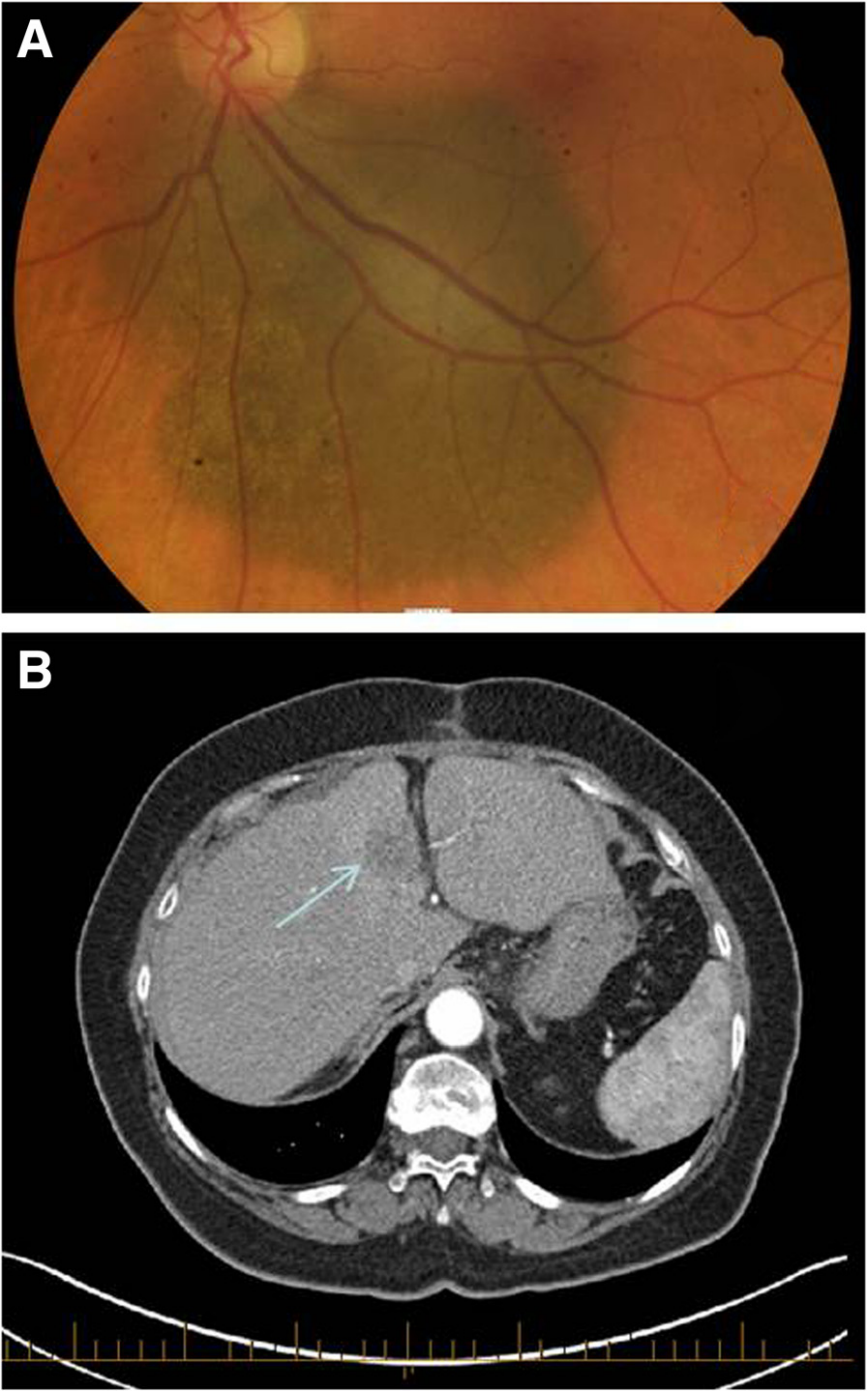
Imaging of patient with germline BAP1 mutation. a. Retinal examination revealed a melanocytic tumor with irregular margins involving the majority of the retina. b. CT of the abdomen prior to surgery identified a 32 mm lobulated lesion in segment 4B of the liver as indicated by the arrow
